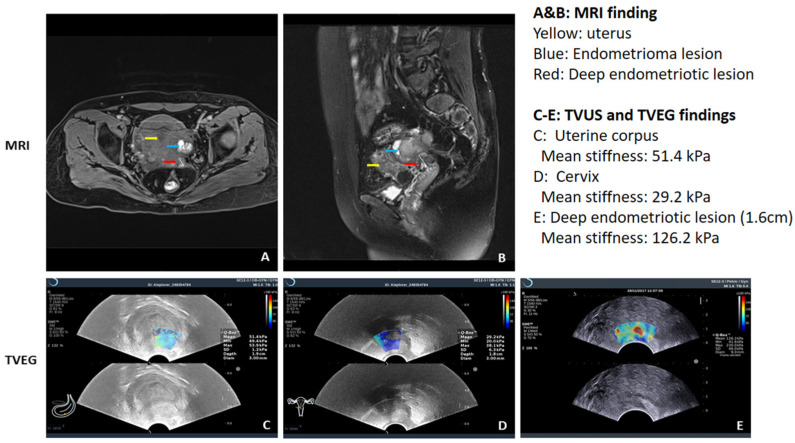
The use of ultrasonic elastography to diagnose deep endometriosis and its consistency with MRI findings. For this patient who complained of severe dysmenorrhea, both MRI (A,B) and a shear-wave ultrasonic elastography (C–E) were used to diagnose deep endometriosis. While the anatomical localization of the deep endometriotic lesion is not as straightforward as MRI, the elastography gave a lesional stiffness value, and in this case the lesion is quite stiff and thus highly fibrotic. Note that for this shear-wave elastography which gives out absolute tissue stiffness value in kilo Pascal (kPa), the color key is reversed, with the blue color depicting the softest while the red color indicating the hardest tissues. More detailed explanation for each figure is given at the upper right panel. (Courtesy of Dr. Ding Ding).
